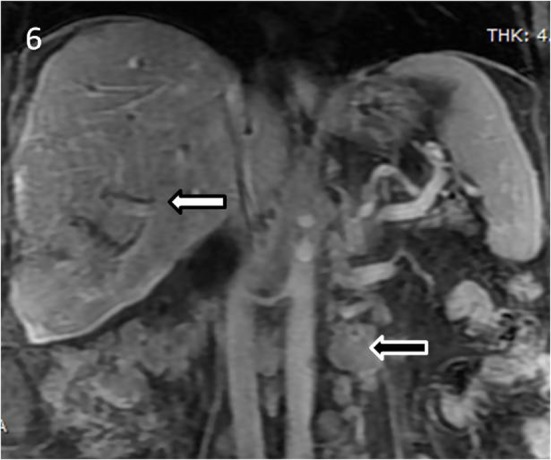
Coronal post contrast MRI T1-weighted fat suppressed sequence showing few prominent (approximately 1 cm in diameter), para-caval and para-aortic lymph nodes were seen in the retroperitoneum.
